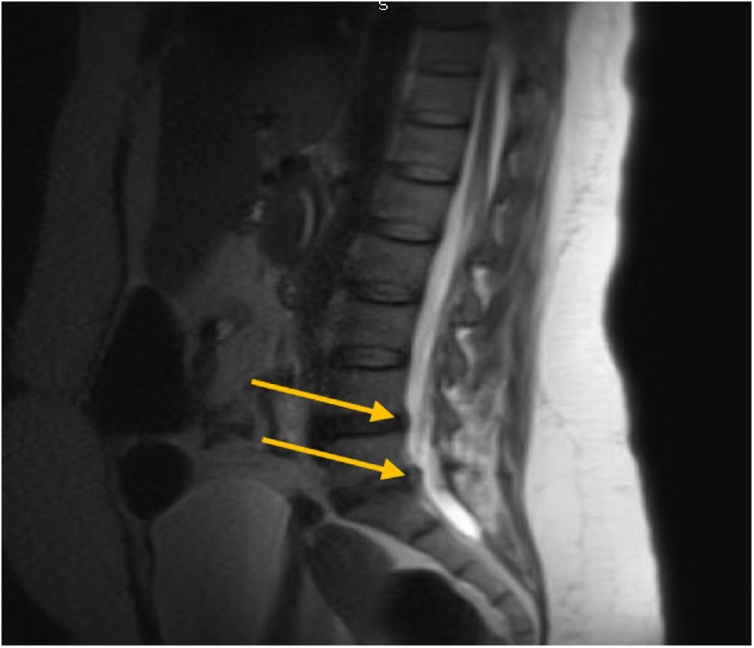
Initial Lumbosacral MRI showing mild impingement on the left L5 and S1 nerve roots.
